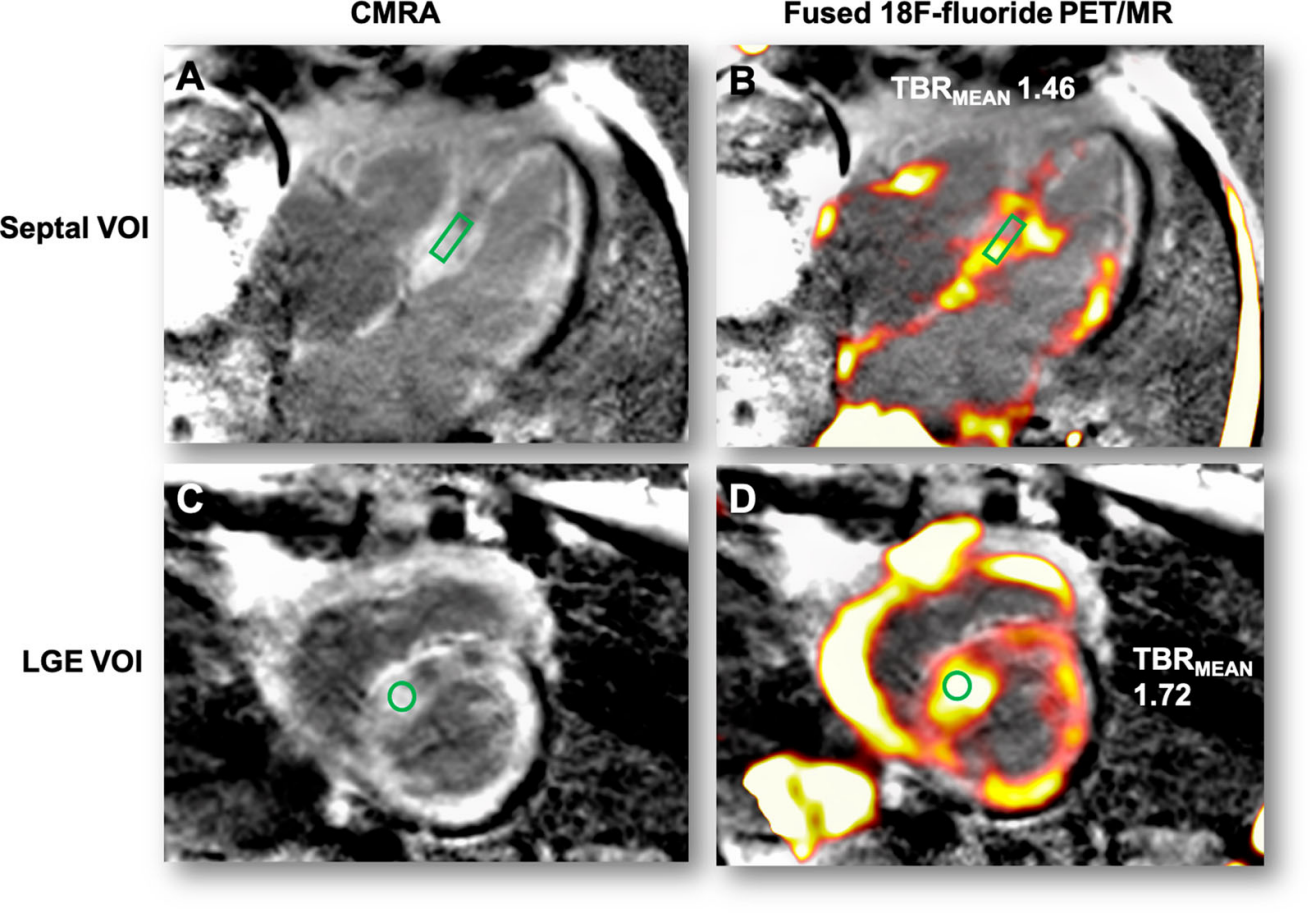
Derivation of myocardial and LGE volumes of interest. Panel A is a 4-chamber view with LGE showing typical myocardial nulling difficulties in a patient with TTR cardiac amyloidosis. The standardized 3 × 15 mm green cylindrical VOI is placed in the septum at the mid-ventricular level. Panel B shows the fused PET/MR with high uptake in the septal and lateral wall of the left ventricle and free wall of the right ventricle (VOI TBR_MEAN_ inset). Panel C shows the LGE image in the short axis with extensive diffuse LGE affecting most of the myocardium with the corresponding short axis view of the septal VOI. Panel D shows the fused PET/MR image of the same slice with diffuse uptake within the left and right ventricular myocardium. Note the intensely high uptake within the most diseased myocardial segment (within the septal VOI) and the difference in TBR_MEAN_ compared to panel B
